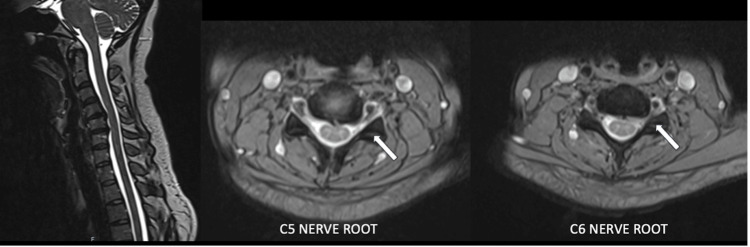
Preoperative MRI of the cervical spine in sagittal (left) and axial views of the C4/C5 (center) and C5/C6 levels (right), demonstrating foraminal stenosis with disc herniation at the left C5 and C6 nerve roots (arrows). MRI: magnetic resonance imaging.
